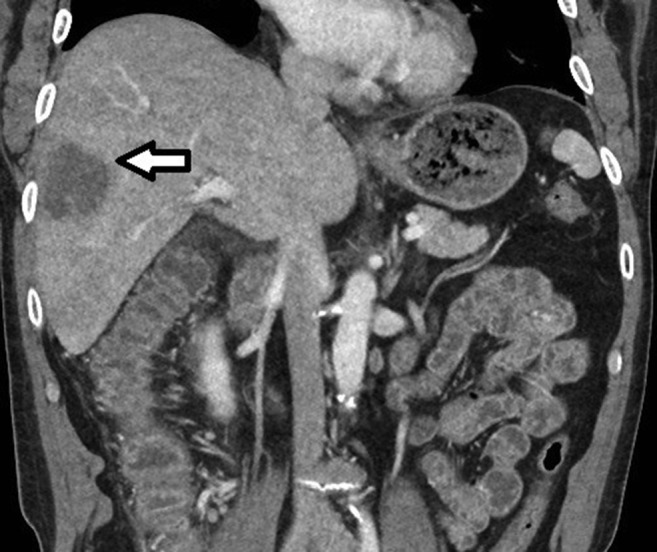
Computed tomography demonstrating amebic liver abscess in the right hepatic lobe.
